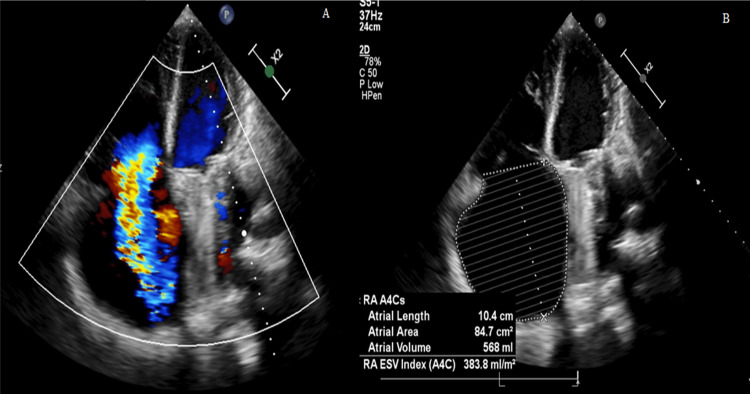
Transthoracic echocardiogram at four chambers view A) Color Doppler of torrential tricuspid regurgitation (vena contracta = 25 mm). B) Right atrium volume measurement.
